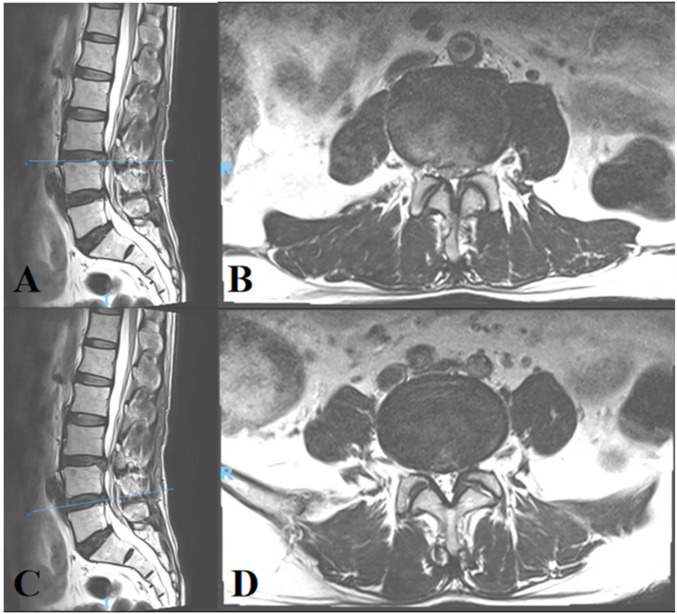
Magnetic resonance imaging (MRI) shows that both L3–4 and L4–5 have lumbar intervertebral disc protrusion. L3–4 sagittal view; axial view of L3–4; L4–5 sagittal view; L4–5 axial view.
